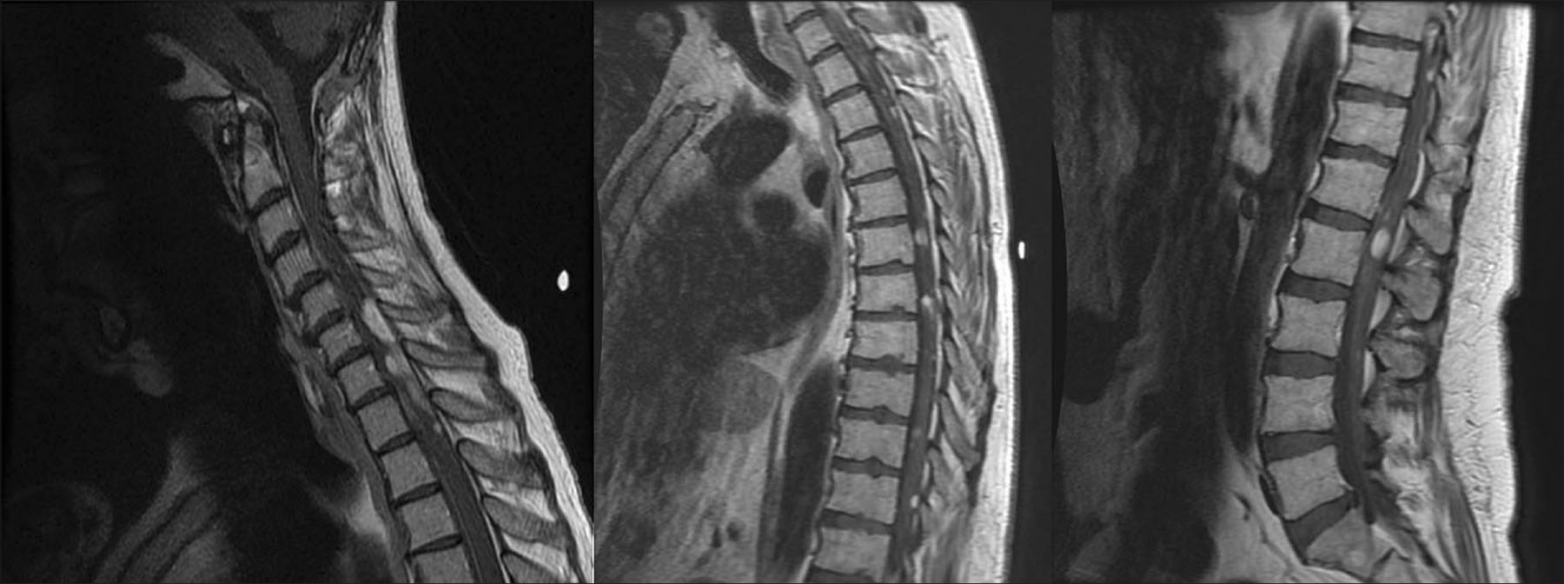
Sagittal T1-weighted MRIs with gadolinium enhancement showing multiple intradural, intra-, and extramedullary metastases
